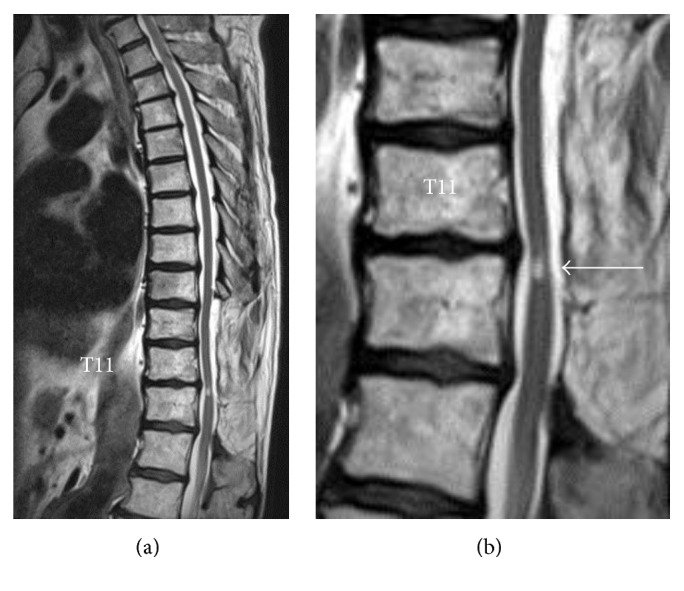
(a) Midsagittal T2-weighted magnetic resonance image of the thoracic spine, 2 years before the HAL intervention and 15 years after the T9–T12 laminectomy. (b) A high-power magnification view of (a), showing a high-intensity change persisting in the decompressed spinal cord at the T11-T12 level (arrow).
